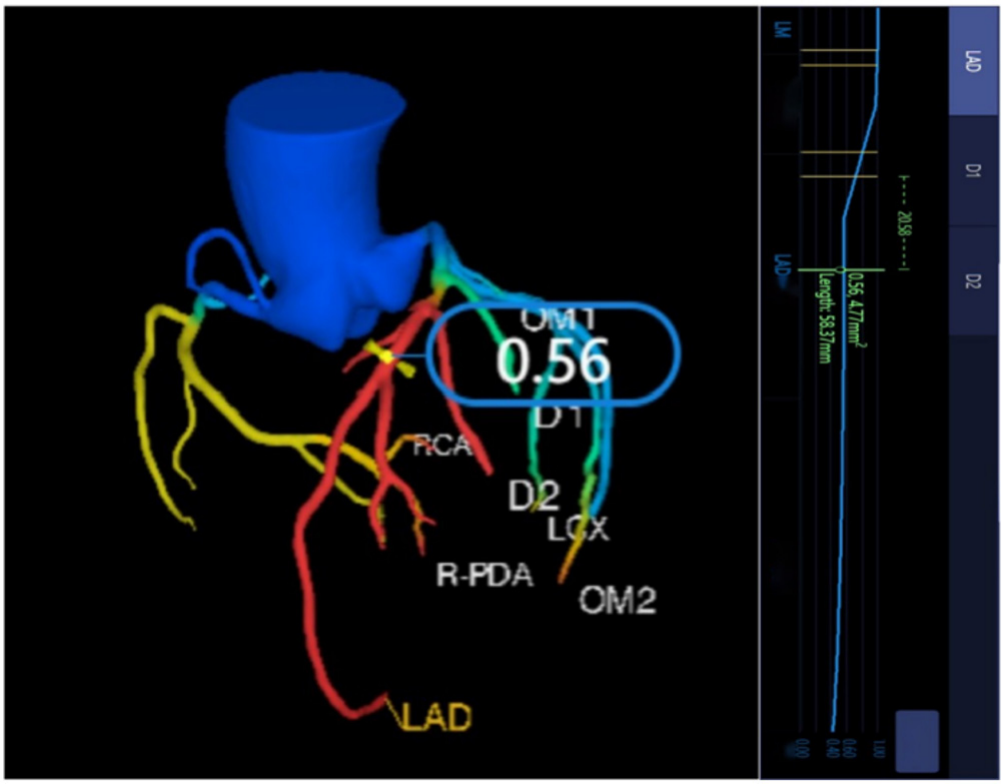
Representative measurement of FFR values on CCTA using an AI software. In the coronary dendrogram displayed on the AI software, the closer the vessel color is to blue, the higher the FFR value, the closer the vessel color is to red, the lower the FFR value. In this representative case, the FFR value is 0.56, measured at the narrowest part of the lesion plaque, about 2–3 cm from the vessel’s origin. FFR: Flow reserve fraction; CCTA: Coronary computed tomography angiography; AI: Artificial intelligence; LAD: Left anterior descending branch; D1: First diagonal branch; D2: Second diagonal branch; LCx: Left circumflex; OM1: First obtuse marginal ramus; OM2: Second obtuse marginal ramus; RCA: Right coronary artery; R-PDA: Right posterior descending branch; mLAD: Middle section of left anterior descending branch.
